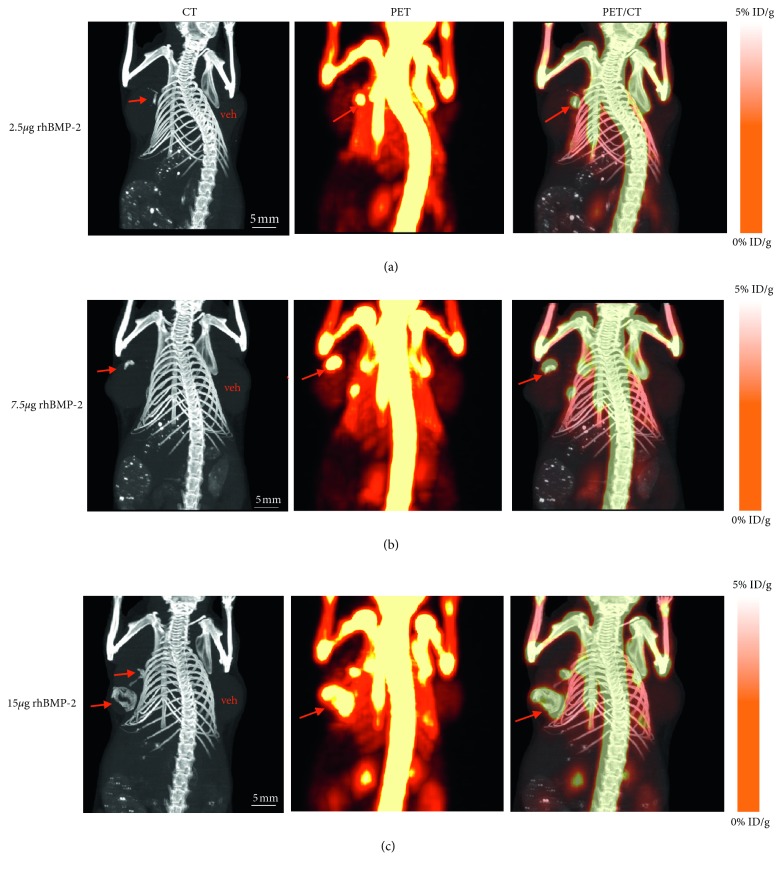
Macroscopic detection of macrocalcifications induced by direct injection of rhBMP-2 into MDA-MB231 syngeneic tumor: both CT imaging and PET imaging indicate that, in comparison to systemic, nonsystemic injection of rhBMP-2 into tumor induced microcalcification in breast tumor was dose dependent, and the uptake of radiotracer (^18^F-NaF) was significantly higher (left tumors in (a)–(c)) than the IP injection of rhBMP-2. There was nondetectable microcalcification or radiotracer uptake by control tumors (right tumors in a–c).
